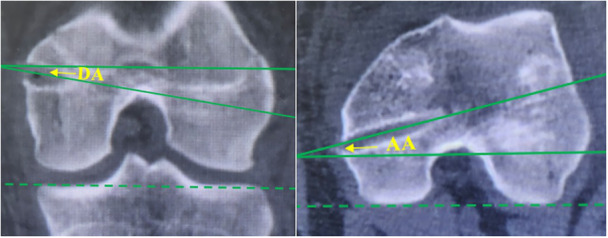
Measurement of DA and AA using post‐operative CT. AA, anterior tilt angle of the bone tunnel; CT, computed tomography; DA, distal tilt angle of the bone tunnel.
